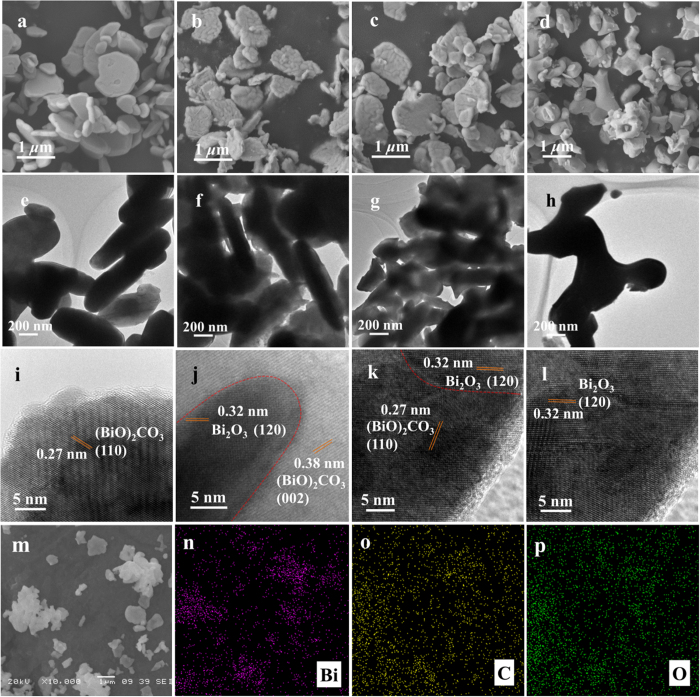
Morphological characterization of the as-prepared samples. (a–d) SEM, (e–h) low-magnification, and (i–l) HR-TEM images of (BiO)_2_CO_3_, BOC-400, BOC-450, and Bi_2_O_3_, respectively. (m) SEM image of BOC-450 heterojunction and the corresponding (n–p) elemental X-ray mapping for bismuth, carbon, and oxygen elements.
